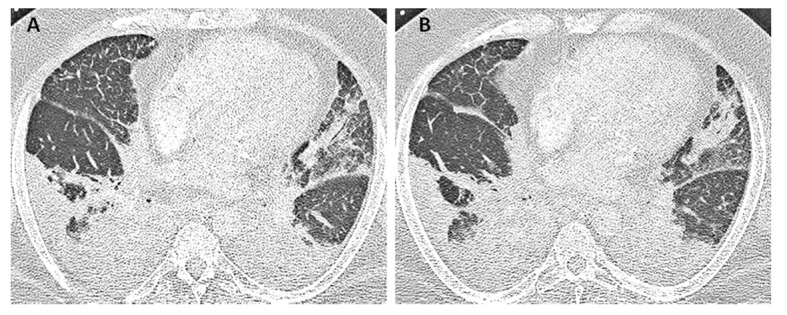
Patient’s CT scan of chest on day 7 (A, B) demonstrated mediastinal lymphadenopathy, diffuses pulmonary nodules, multifocal consolidations in right upper and lower lobe, lingular lobe, and left lower lobe as well as bilateral pleural effusion.
